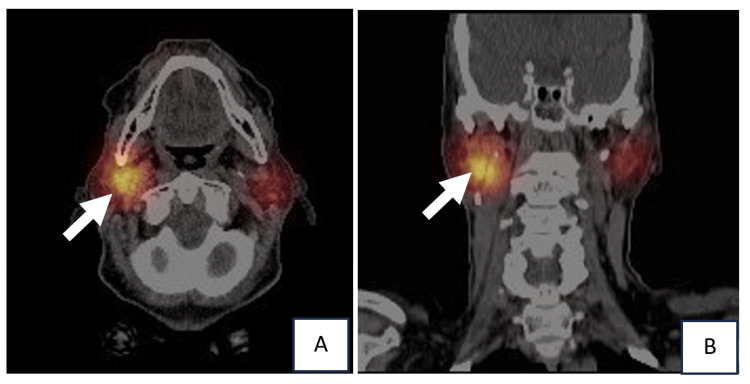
(A) Axial and (B) coronal fused SPECT/CT images demonstrate focal abnormal I-131 uptake within the right parotid gland (white arrow). SPECT: single-photon emission computed tomography; CT: computed tomography
